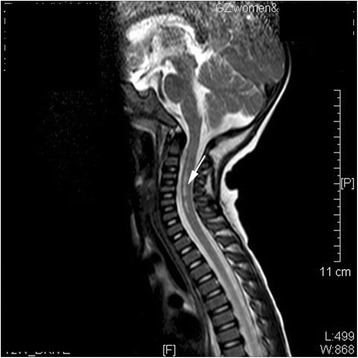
High MRI signal in C2-C7
